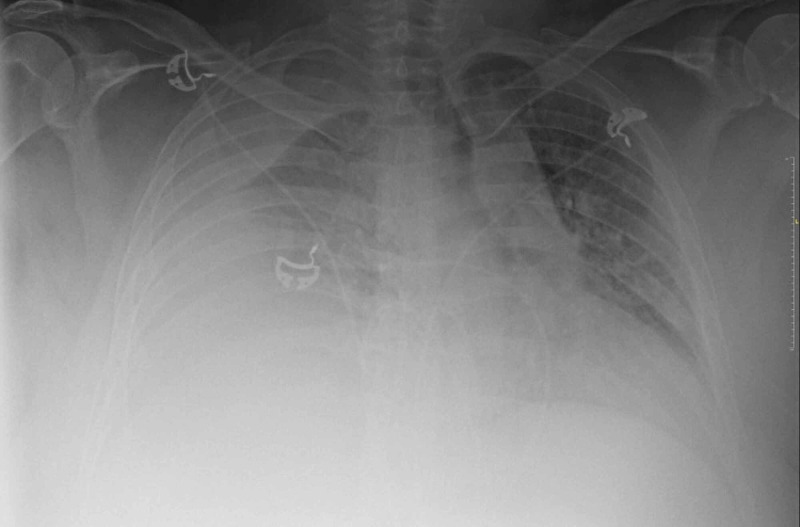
Chest X-ray Anterior-posterior (AP) view showing increased large right pleural effusion with aeration loss of much of the right lung as well as accompanying leftward mediastinal shift.
